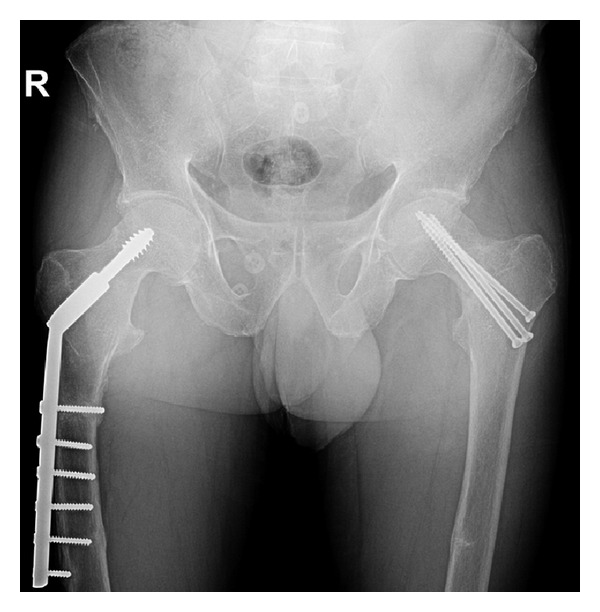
Anteroposterior X-ray showing bilateral hip fracture that underwent screwing.
